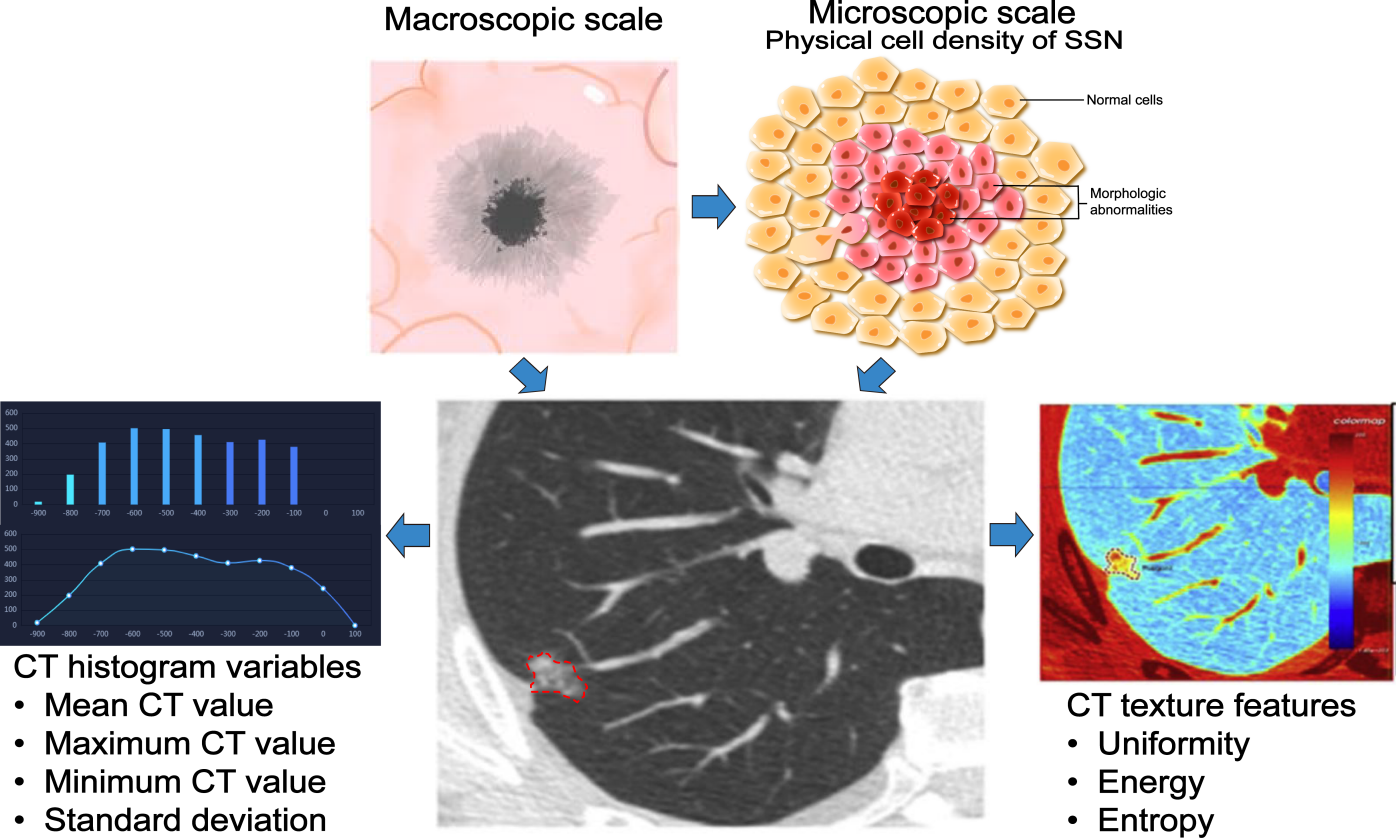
Application of three-dimensional measurement index in SSN. The performance of the SSN is determined by the physical cell density and atypia, and the CT value can reflect the physical cell density of the SSN in a macroscopic form. With CT manifestations, SSNs growth can be predicted based on CT histogram (mean CT value, maximum CT value, minimum CT value, standard deviation of CT value) and texture features (entropy, uniformity, and energy), resulting in better management of nodules.
